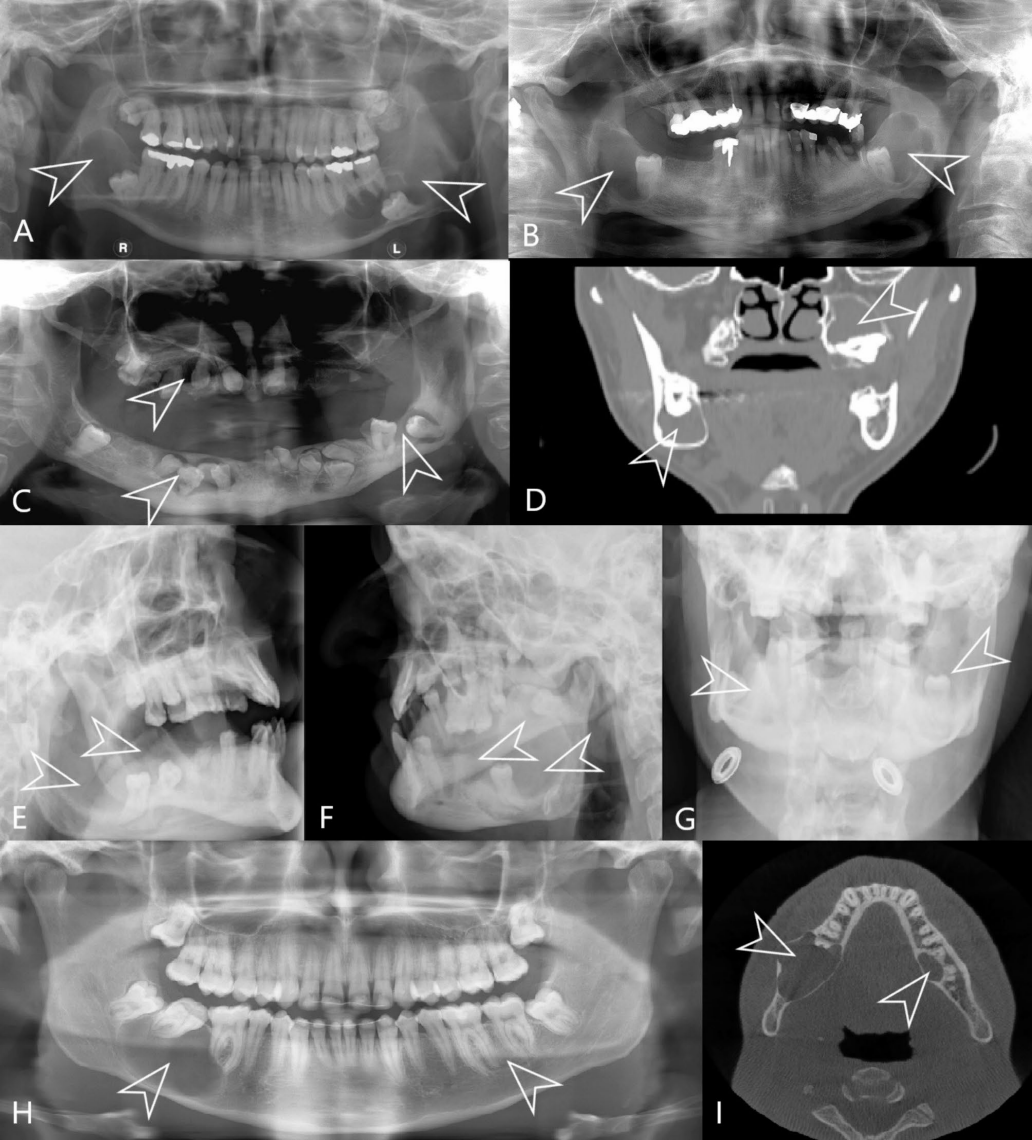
Examples of multiple non-syndromic developmental odontogenic cysts. Locations of the cysts are marked with white arrows. A, B – Panoramic X-rays of bilateral dentigerous cysts (DCs), C – Panoramic X-ray of multiple non-syndromic DCs, D - Computed tomography of non-syndromic DCs of the maxilla and mandible. E, F, G – X-rays of the head of a patient with bilateral non-syndromic odontogenic keratocysts. H, I – Panoramic X-ray and Cone-beam computed tomography of a patient with bilateral orthokeratinizing odontogenic cysts
